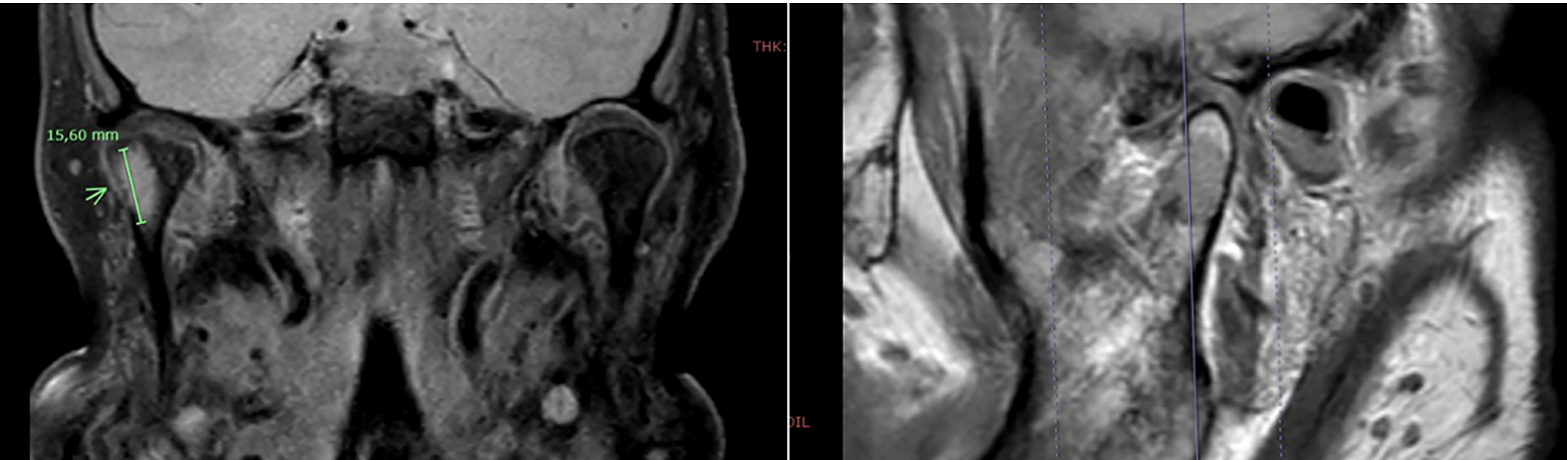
Coronal (right) and sagittal (left) MRI slices showing an eccentric lesion in the right mandibular condyle.
